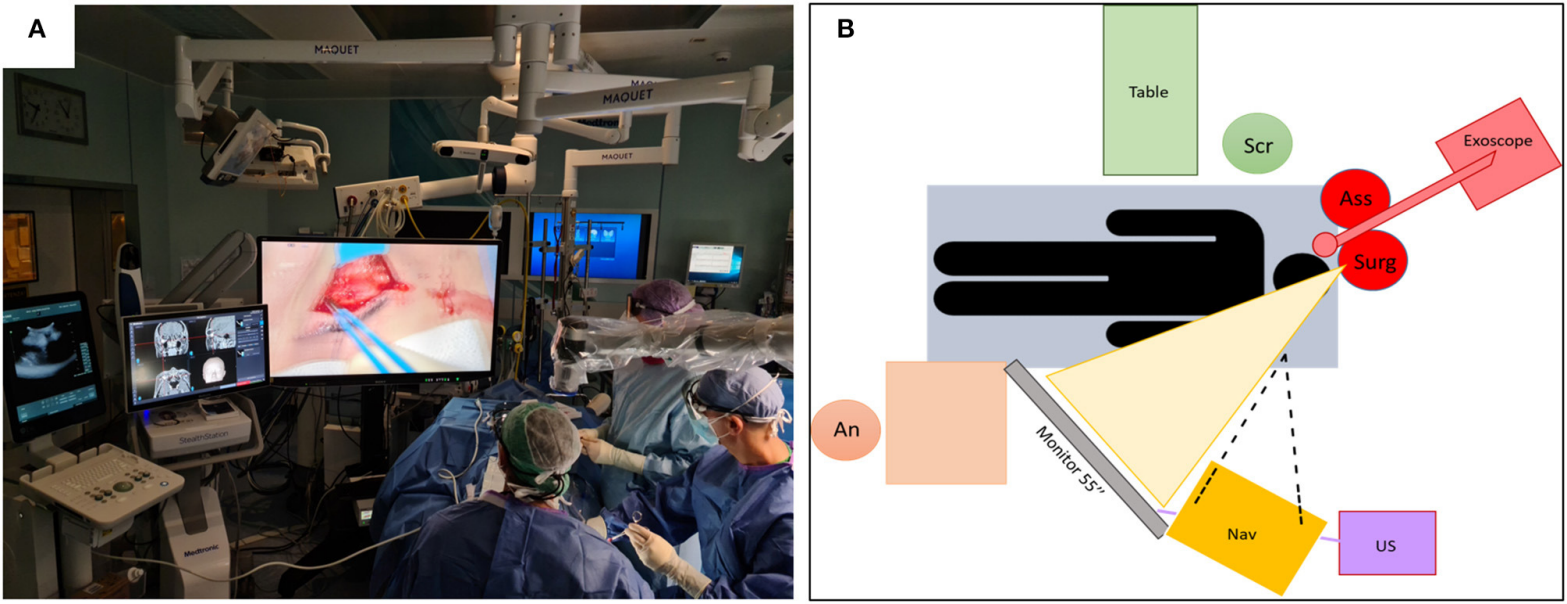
Placement of the technologies in use (4K-3D camera, 55-inch monitor, navigator, and ultrasound) and staff within the OR during surgery for right transpalpebral approach (A) and scheme (B). Note the comfortable position of the surgeon who can operate while looking at the monitor with the head in an upright position.
